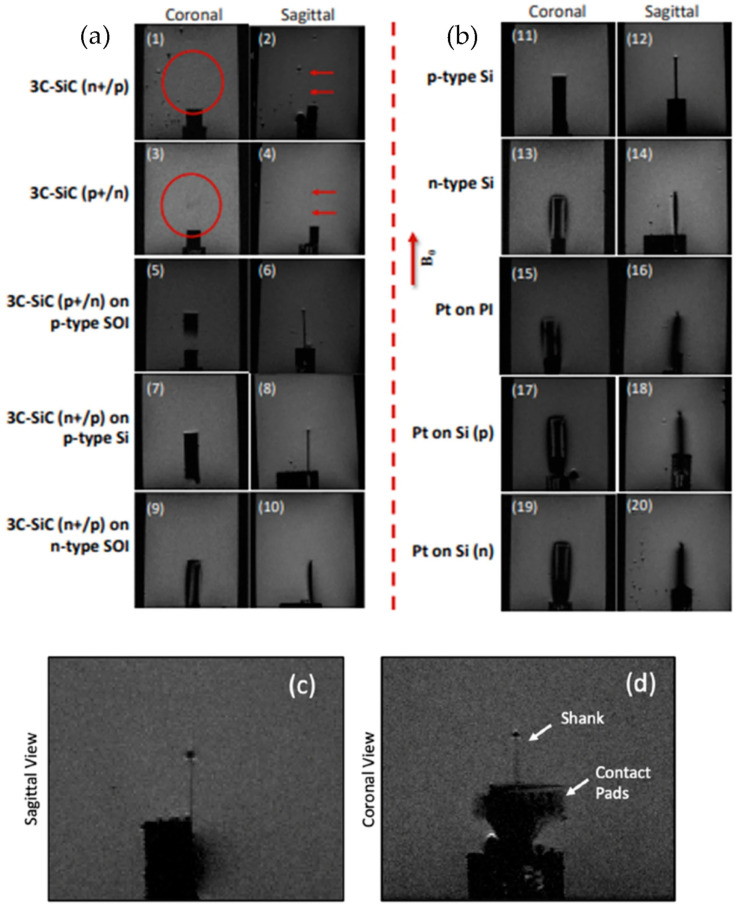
7T MRI images of different semiconductor materials: (a) (1, 2) 3C-SiC (n^+^/p) is almost invisible in the scan. (3, 4) 3C-SiC (p/n^+^) similar to (1, 2). (5–8) Minimal artifacts, yet visible samples, for 3C-SiC on p-type SOI and Si substrates. (9, 10). Artifacts and samples are visible for 3C-SiC on n-type SOI substrate. (b) (11–14) Artifact differences between n- and p-type Si substrates. (15–20) Severe image artifacts for all platinum samples. (c,d) The sagittal and coronal views of the 3C-SiC probe, showing a low level of artifacts except at the tip of the device and the area where the gold bond pads are located, which would not be within the tissue [85]. The red circles and arrows in (1) to (4) show the location of the probe which is barely visible in the images.
